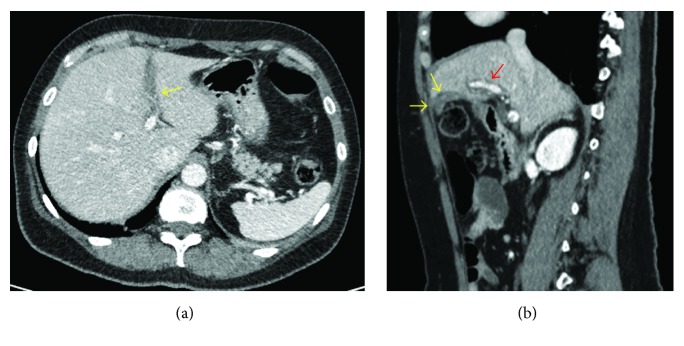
Abdominal axial (a) and sagittal (b) CT scan showing resolution of falciform ligament thrombosis (yellow arrows) with patent left portal vein (red arrow).
